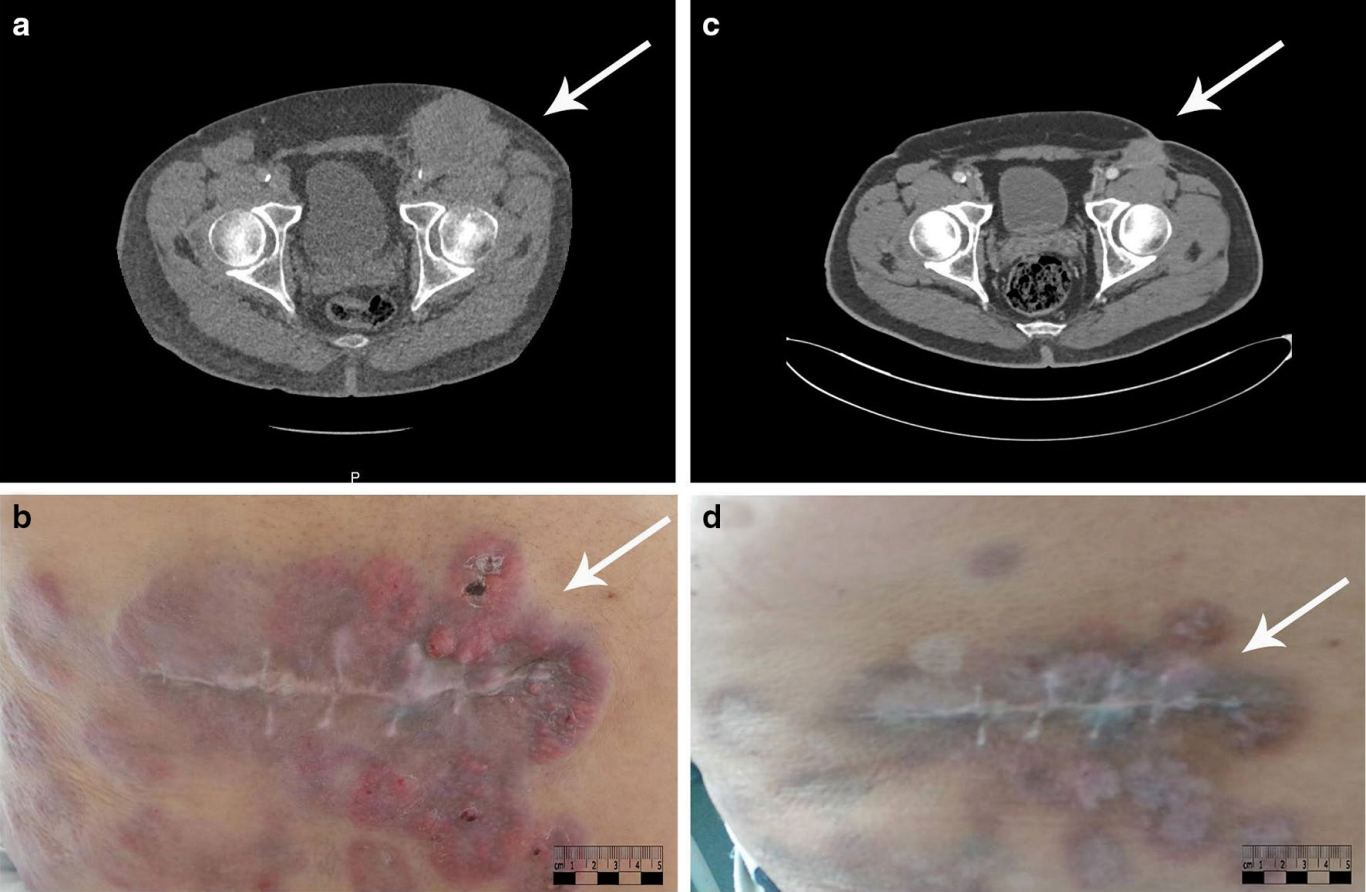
Computed tomography (CT) and macroscopic images of the inguinal lesion before and after 3 cycles of chemotherapy. a CT scan shows a subcutaneous metastatic melanoma lesion (*arrow*) of 76 mm × 63 mm in the left inguinal area before chemotherapy. b Cutaneous metastatic melanoma lesions (*arrow*) were nodular and inflammatory before chemotherapy. c CT scan shows that the size of the subcutaneous metastatic melanoma lesion (*arrow*) decreased to 31 mm × 35 mm, with a reduction of 48%, after 3 cycles of chemotherapy. d Cutaneous metastatic melanoma lesions (*arrow*) exhibited massive shrinkage, leaving a fibrotic quality of the skin, after 3 cycles of chemotherapy
